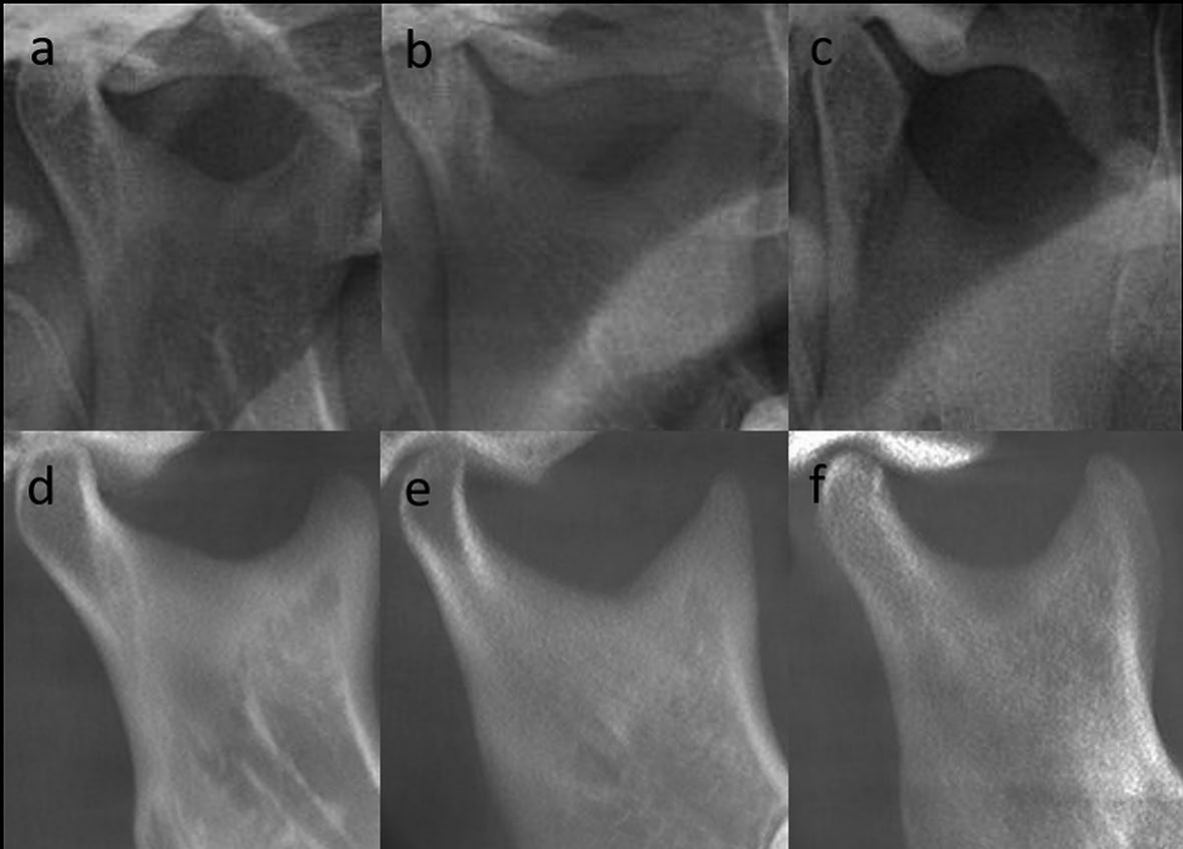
The cropped panoramic image of the SN (a showing sloping shape, b showing wide shape, c showing round shape); Cropped CBCT reformatted panoramic image of the SN (d showing sloping shape, e showing wide shape, f showing round shape).
